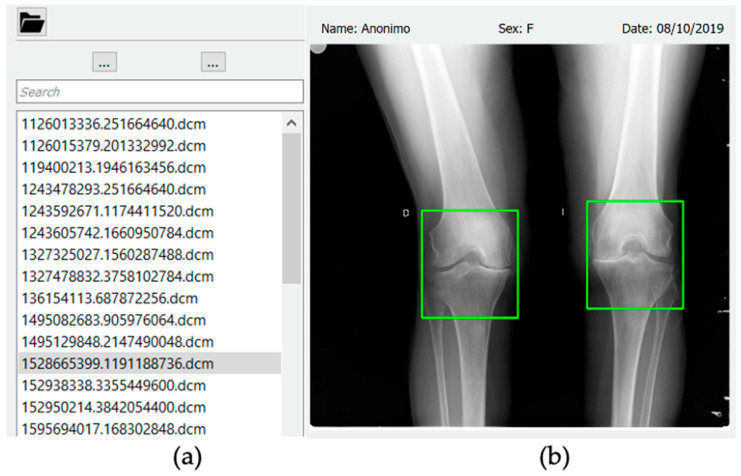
(a) Navigation panel, that allows selection of the image to process (*.dcm files). (b) The X-ray image panel where the region of interest is selected.
